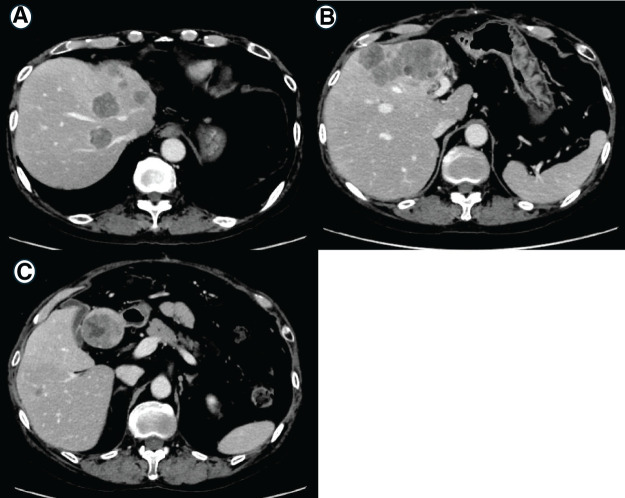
CT imaging obtained 2 months after surgery (A–C) Contrast-enhanced CT showing multiple new hepatic metastases indicating early recurrence following hepatic resection. These findings prompted the initiation of systemic chemotherapy.
